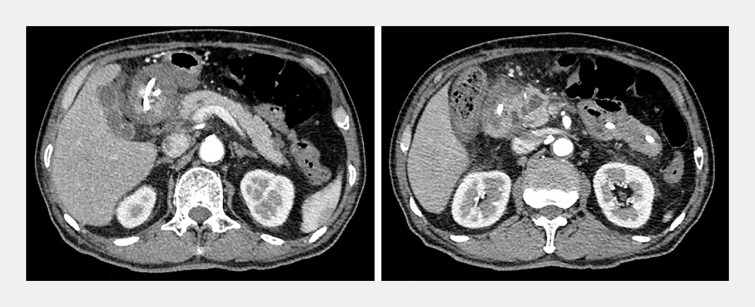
Abdominal contrast-enhanced computed tomography revealed edema and thickening of the duodenal wall, fluid accumulation in the groove area, and enlargement of the pancreatic head with a pseudocyst.
